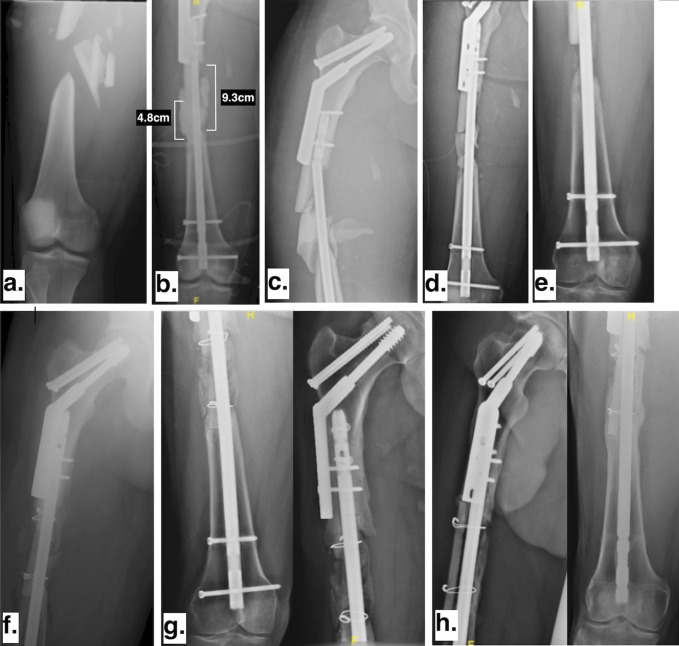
Case 3. (A) Injury radiograph. (B) Radiograpg showing initial fixation with intramedullary nail and cement spacer, proximally distal part of sliding hip screw can be seen. (C) Radiograpg showing failure of intramedullary nail at the distal end of sliding hip screw. (D) Radiograpg showing revision intramedullary nail and placement of new cement spacer. (E) Radiograpg showing second stage of induced membrane technique with placement of iliac crest autograft. (F) Radiograpg showing revision of nonunion with repeat second stage of induced membrane technique, this time with addition of fibular strut allograft. (G) Radiograpg showing six months after placement of fibular strut allograft. (H) Radiograpg showing fifteen months after placement of fibular strut allograft.
